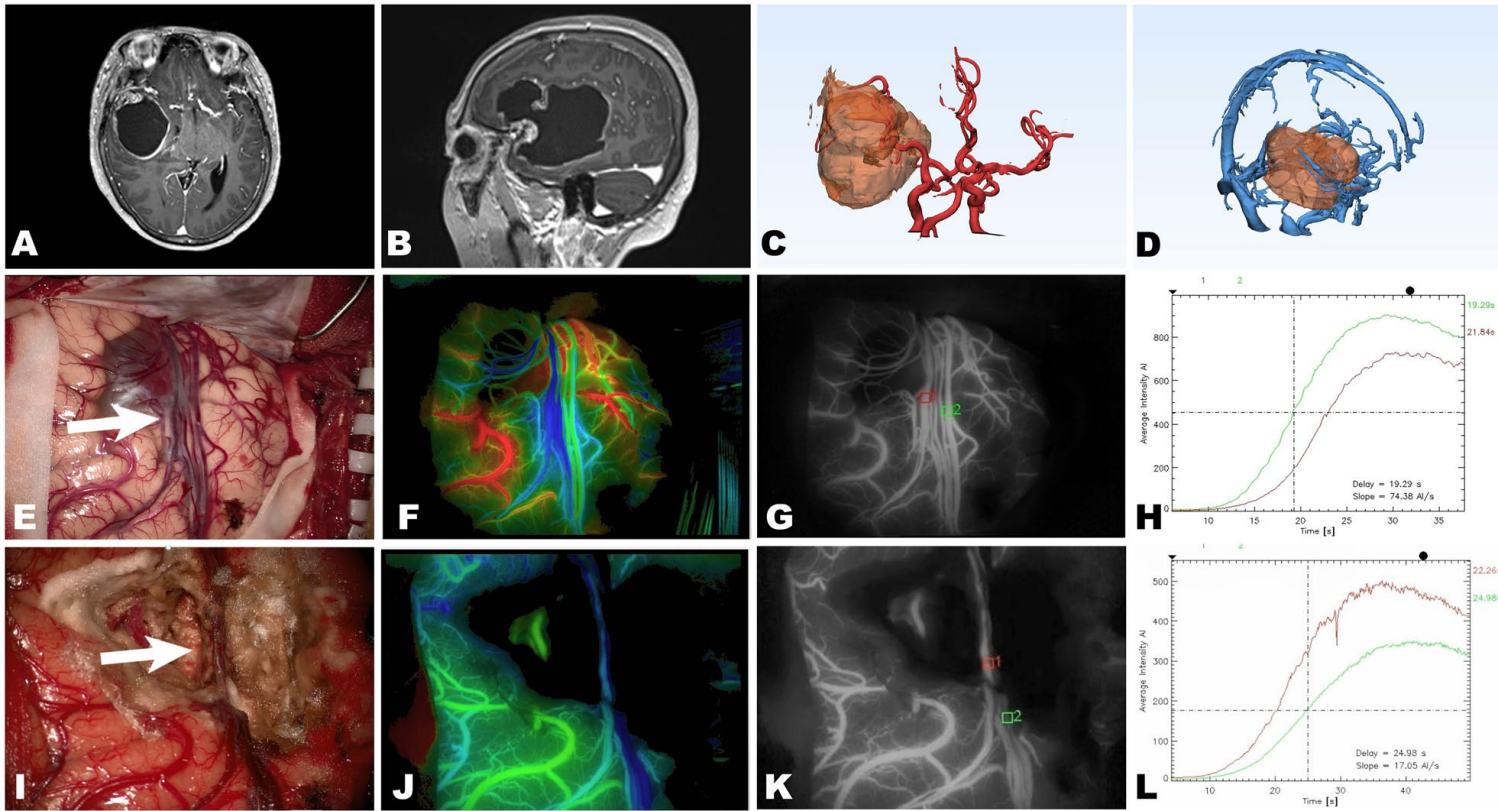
A patient with a large cystic mass in the right frontotemporal lobe underwent surgery. A T1-weighted axial and B T1-weighted sagittal MRI with contrast demonstrated a cystic mass located at the right frontotemporal lobe. C 3D reconstruction imaging showed the right middle cerebral artery was displaced and encased by the mass. D 3D reconstructed images also revealed the encased sylvian vein. E, I The mass was removed via a pterional approach and the sylvian vein (arrow) was preserved, F–L ICG-VA integrated with FLOW 800 confirmed the patency of the sylvian vein, and semiquantitatively analysed the blood flow of the protected vein
